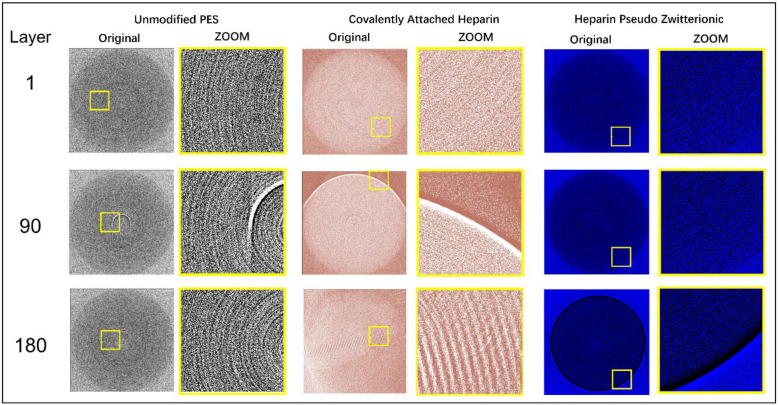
CT slices of membrane layers (top, middle, and bottom).
